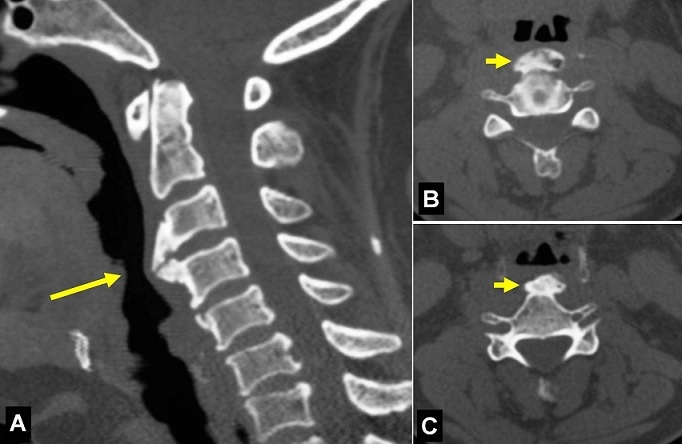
Cervical computed tomography scan (body windows) on sagittal (A) and axial (B and C) views revealing an important anterior cervical osteophytosis (arrows) at C3-C4 vertebral level causing compression of the upper airway and the pharynx
